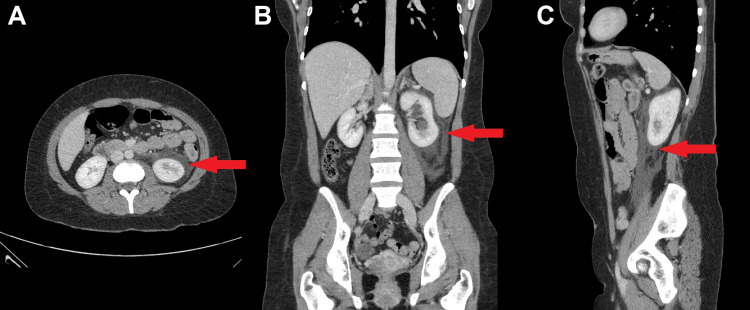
Axial (A), coronal (B), and sagittal (C) views of CT of the abdomen and pelvis with contrast showing left perinephric inflammatory changes and fluid tracking (arrows), suggestive of a urine leak extending down the retroperitoneum
